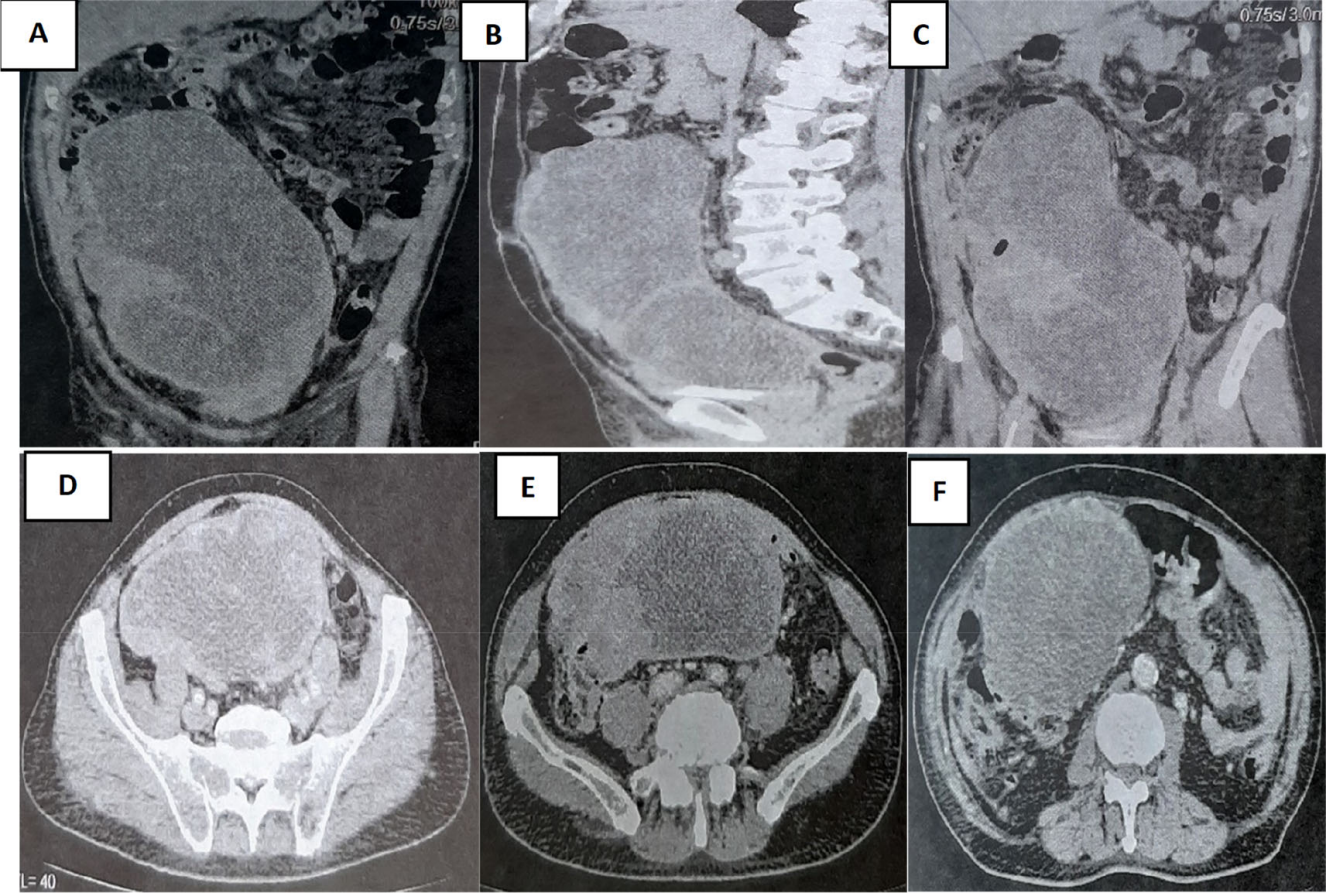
CT scan showing tumor mass.
